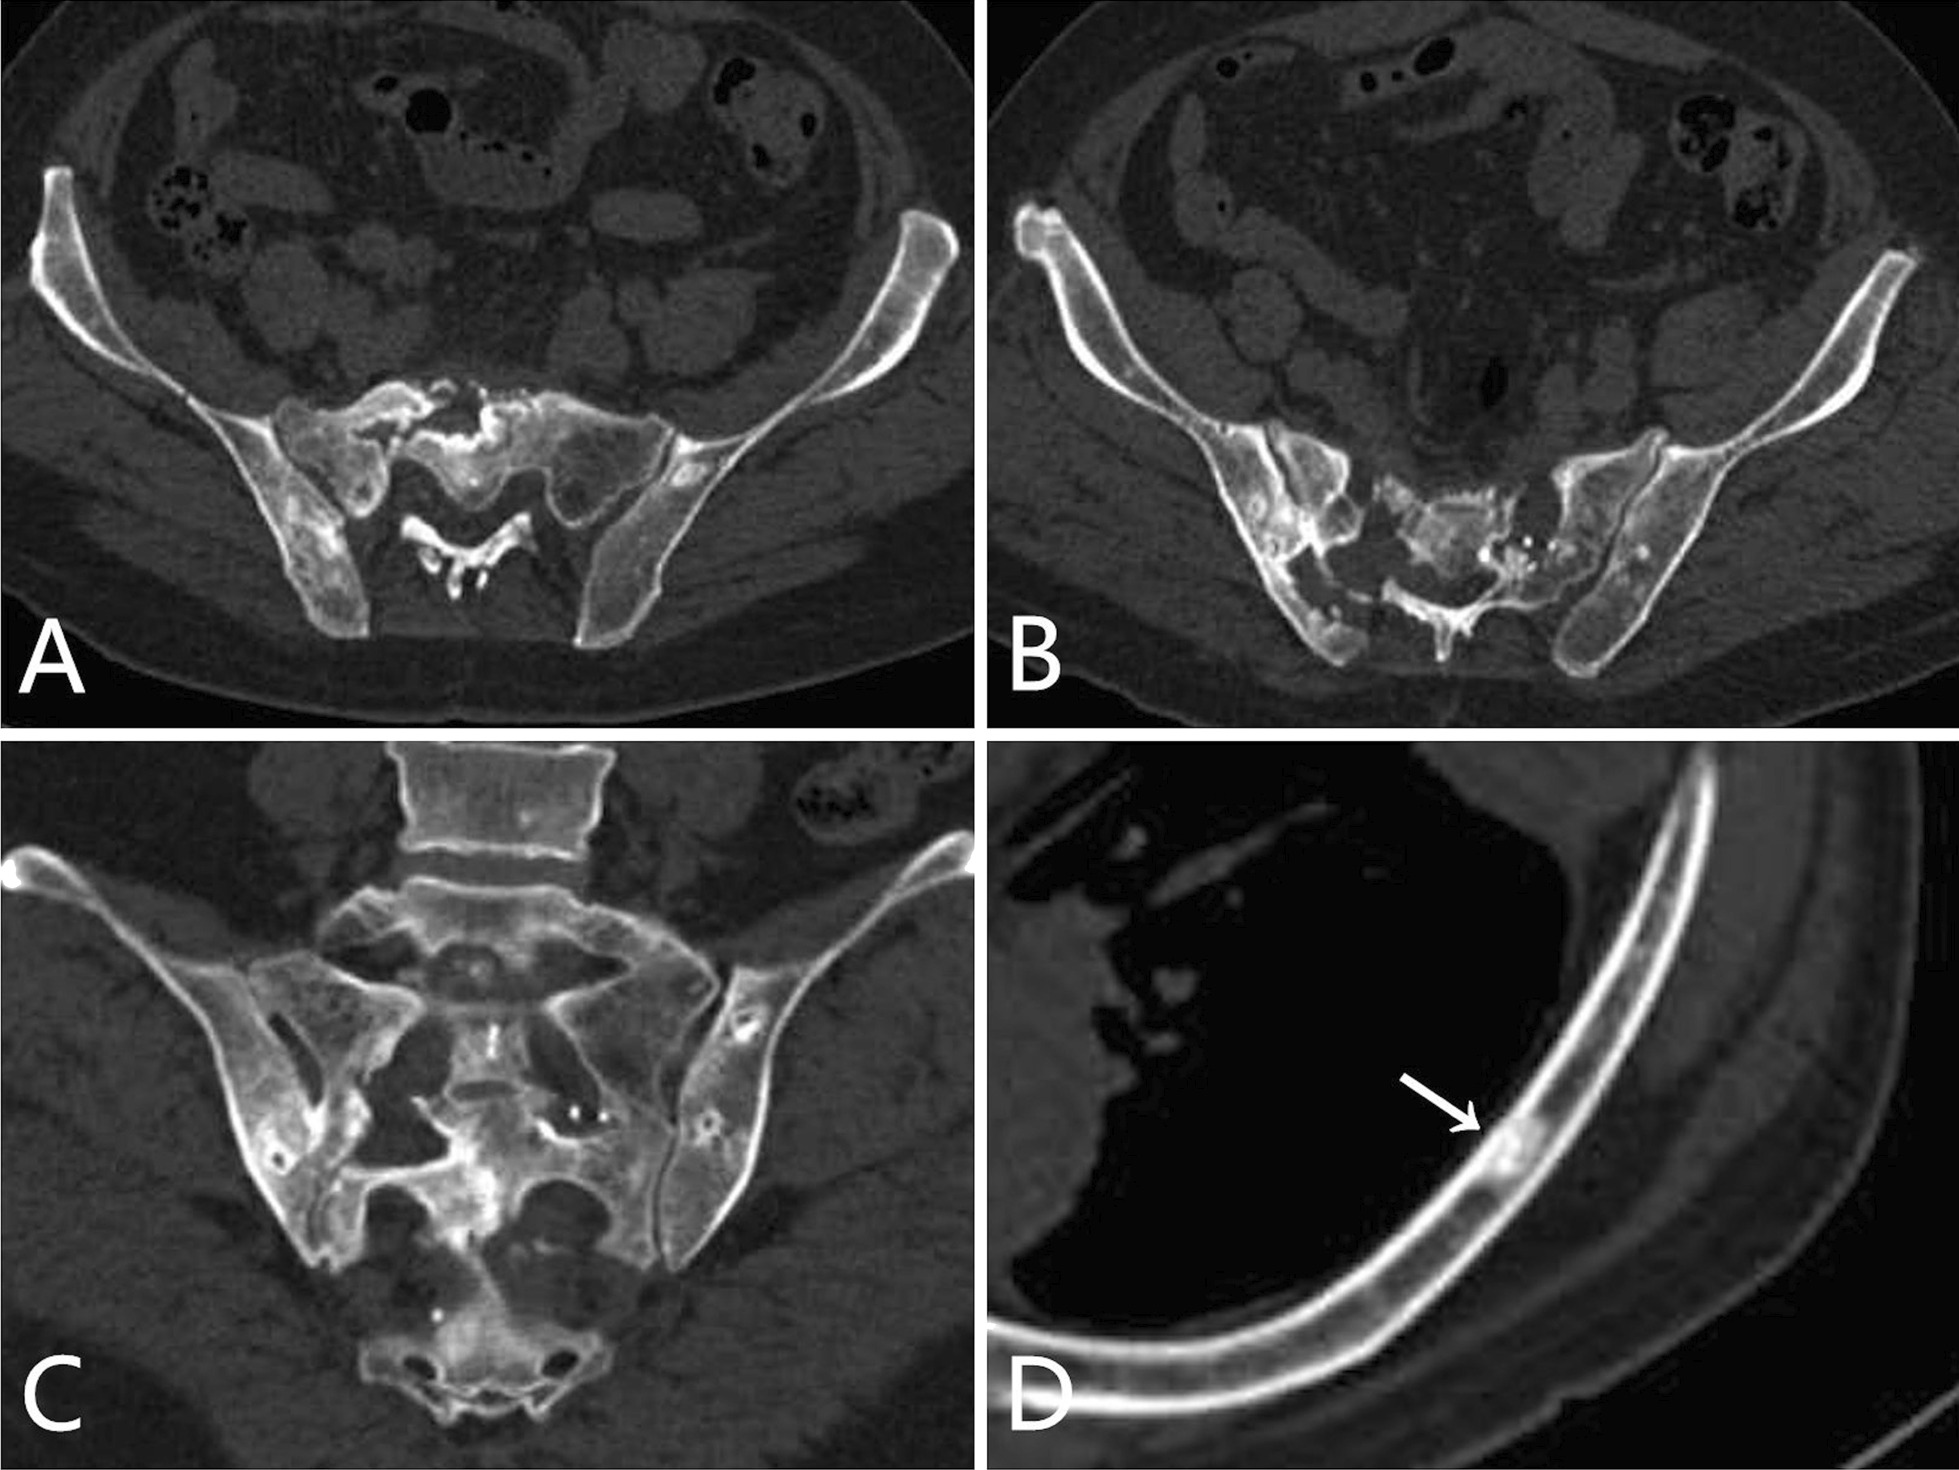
CT images in a 52-year-old man with pelvis, spine, and ribs involved. A–B axial pelvic CT images and C coronal reconstruction pelvic CT image show mixed osteolytic and osteosclerotic lesions in the right iliac bone and the sacrum with the destruction of the sacroiliac joint, as well as multiple lytic lesions with sclerotic margins in the right iliac bone. D Axial chest CT image demonstrates focal osteosclerotic lesion of the ninth left side rib without expansion (arrow)
